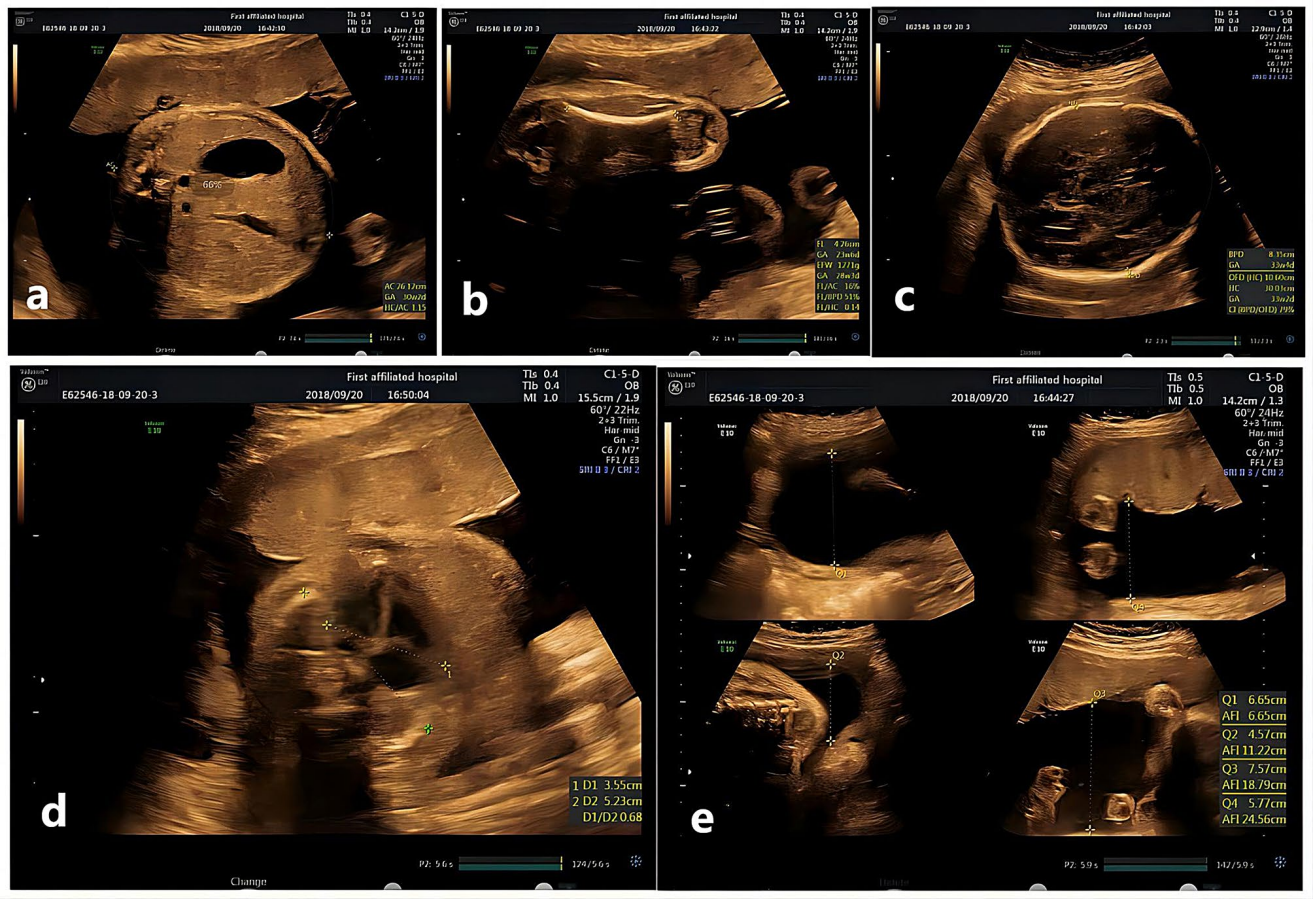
Sonographic features of the fourth fetus with DYNC2H1 compound heterozygous variants. (a) The abdominal circumference (AC) was equal to the length of 30 + weeks of gestation (26.12 cm). (b) The femur length (FL) was equal to 24 weeks of gestation (43 mm). (c) The head circumference (HC) was equal to the length of 33 + weeks of gestation (30 cm). (d) Thoracocardiac ratio:0.68 and thoracic stenosis. (e) Polyhydramnios (depth 25.6 cm)
